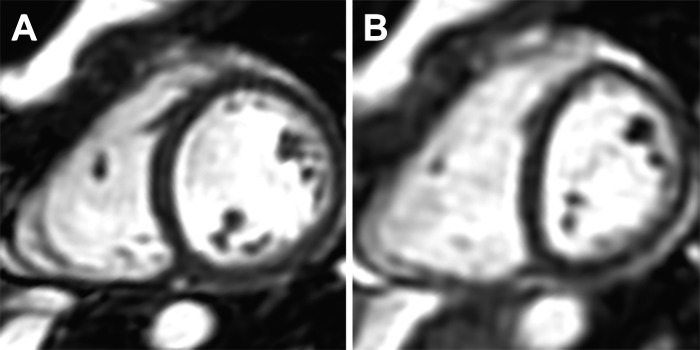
Cardiac magnetic resonance images of a healthy control subject in the left ventricular short-axis plane at end expiration during normoxia (*A*) and hypoxia (*B*), demonstrating septal flattening associated with hypoxia-induced pulmonary arterial hypertension.
